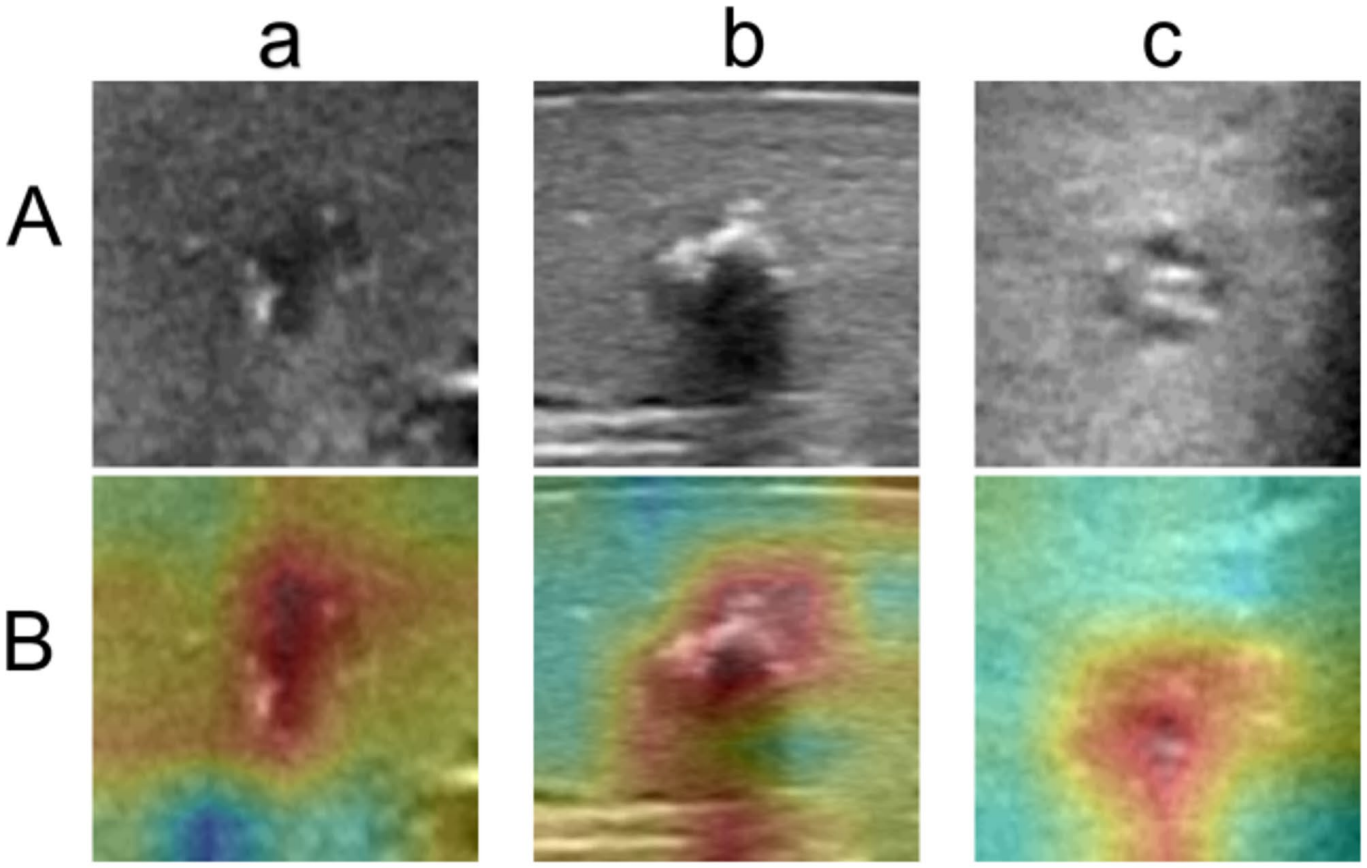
The ultrasound images of thyroid calcified nodules from three different patients (a, b, and c) are presented, along with their corresponding heatmaps
